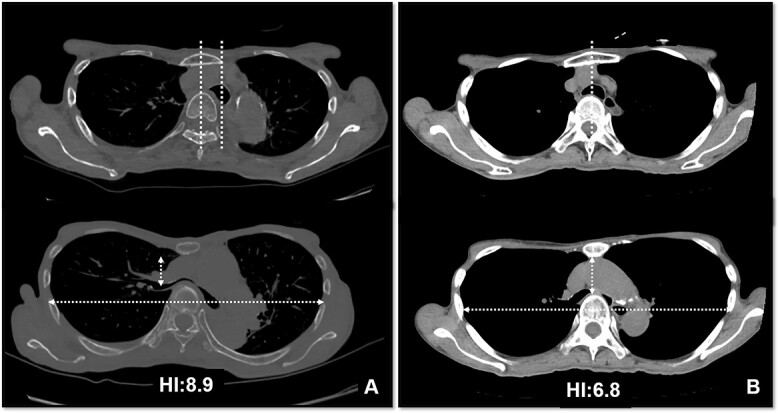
Pre- (A) and postoperative (B) CT findings: the Nuss procedure improved the chest wall and right main bronchus stenosis, and reduced HI from 8.9 to 4.6.
